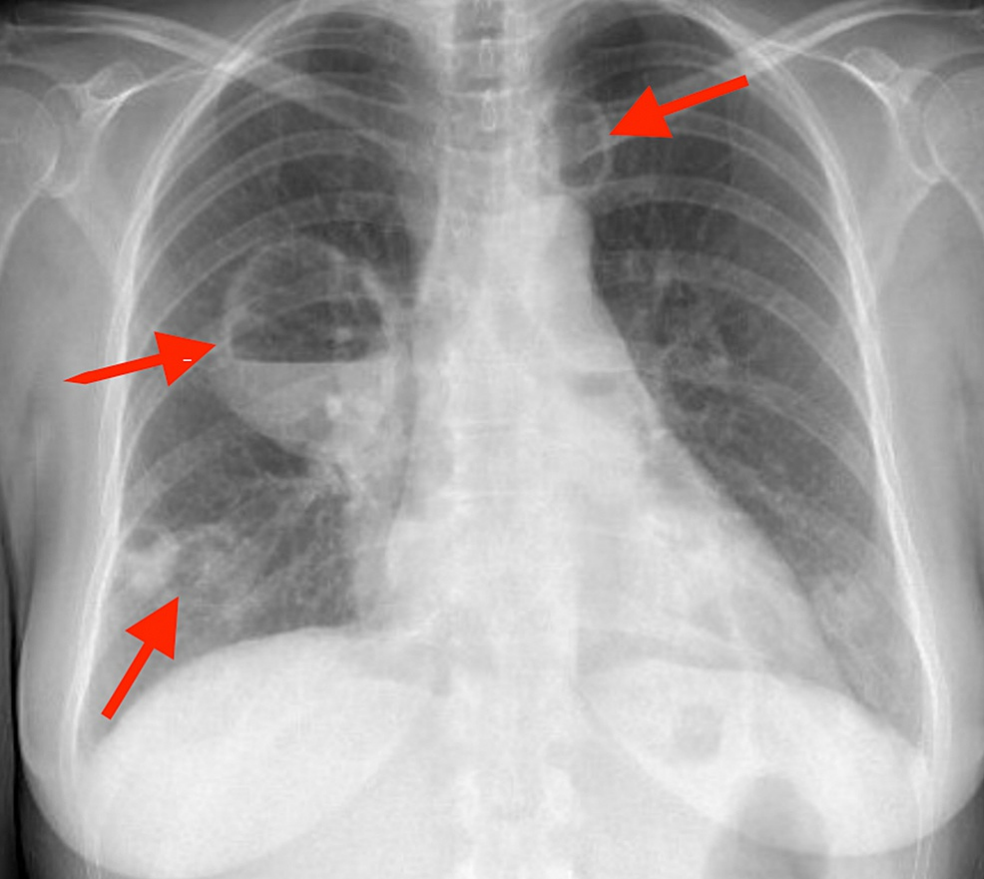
Chest x-ray showing multiple bilateral non-cavitating and cavitating masses (red arrows)
